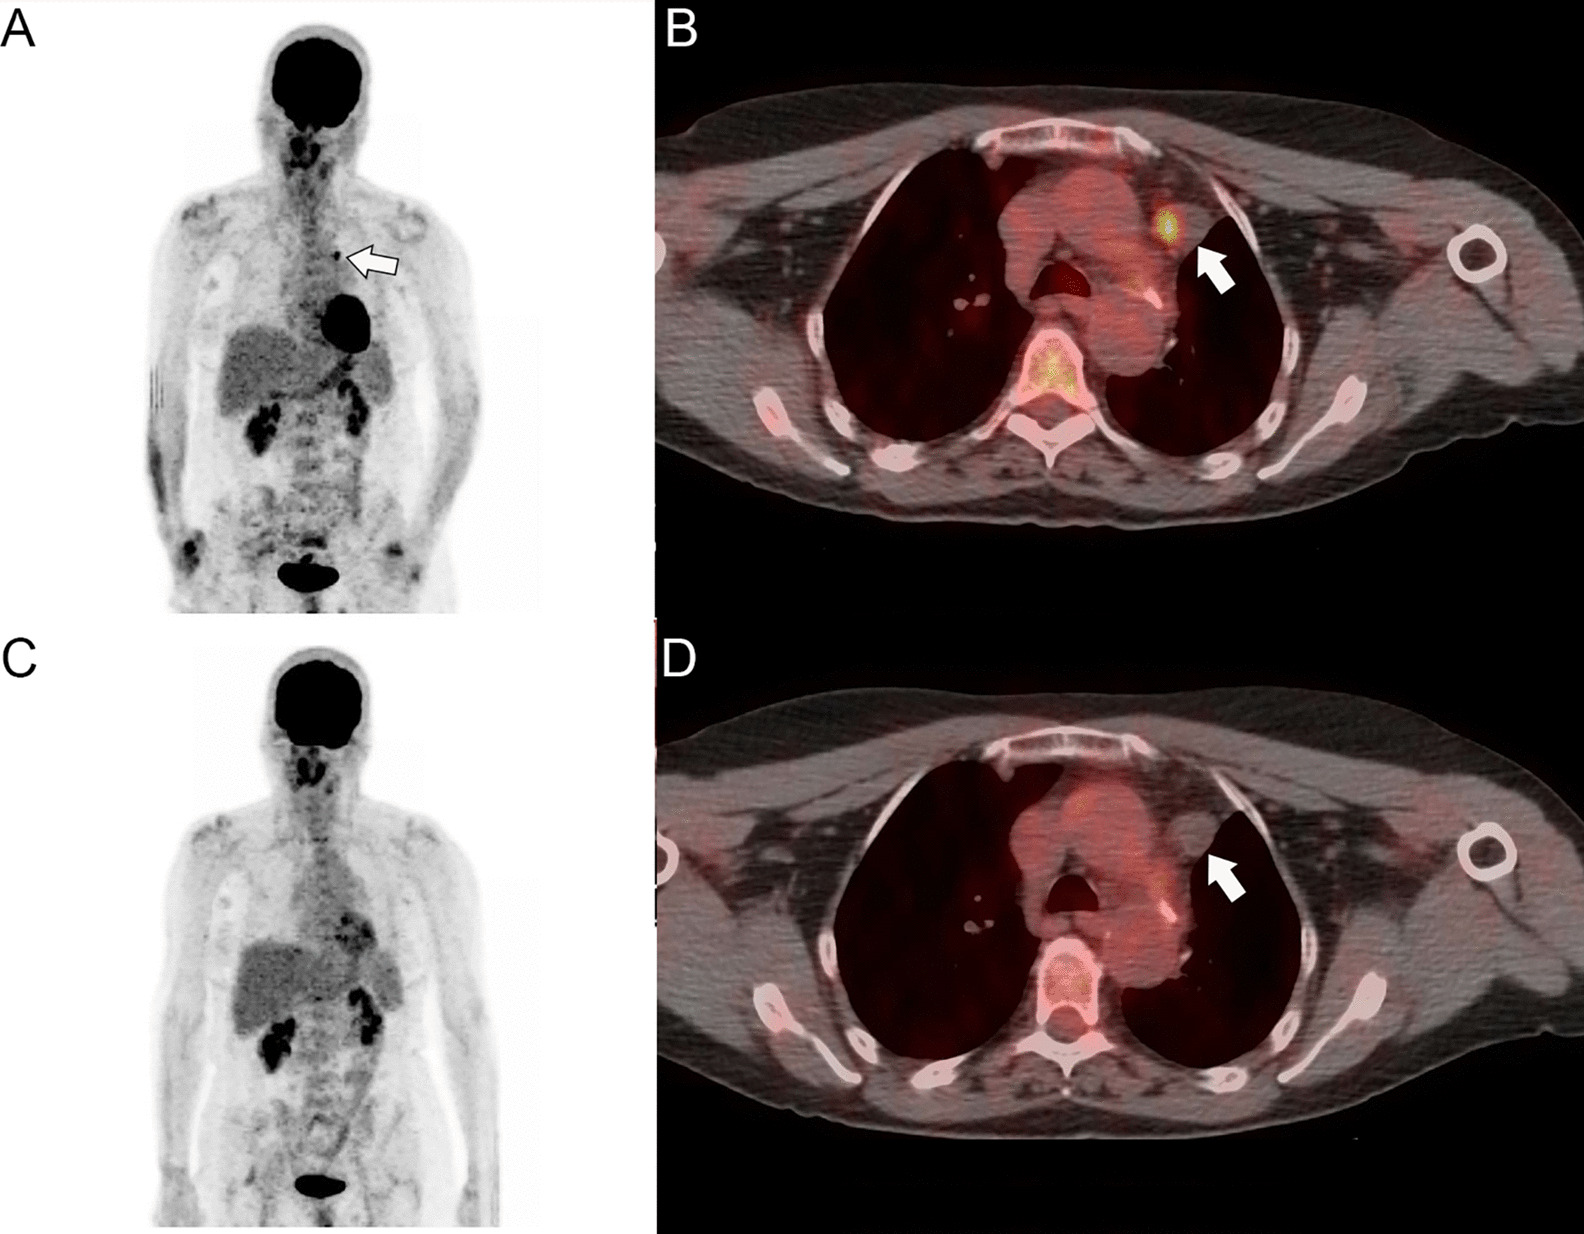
The PET (a) and PET/CT (b) performed at the time of recurrence in September 2015 demonstrate a metabolically active subaortic lymph node metastasis (arrows). The subsequent PET (c), performed approximately 1 year after commencing treatment with pembrolizumab, did not demonstrated any metabolically active disease, and the PET/CT (d) confirms no metabolic activity is associated with the prior subaortic lymph node (arrow)
